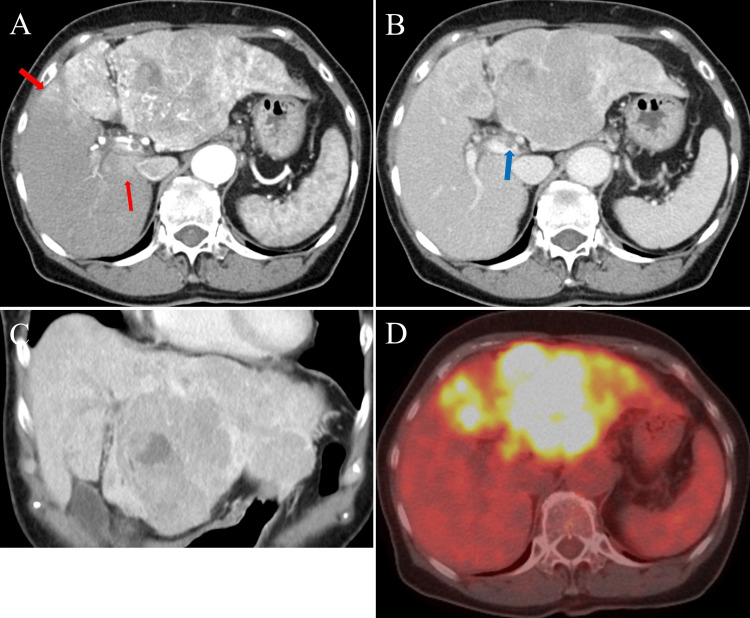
CT images at initial diagnosis A-C: CT images showed an irregularly cirrhotic liver with a large, 150-mm diffuse hepatocellular carcinoma (HCC) replacing the left lobe. The tumor appeared hyperattenuated in the arterial phase and hypodense in the portal venous phase. Multiple smaller HCC lesions were observed in the right lobe (shown by the red arrow). A tumor thrombus was visible in the left branch of the main portal vein (shown by the blue arrow). No lymph node involvement or distant metastases were identified. D: 18F-Fluorodeoxyglucose positron emission tomography/CT showing metabolic activity in the tumor-affected regions identified on CT.
